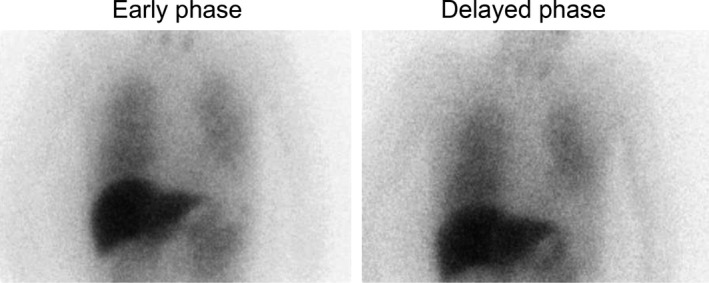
Results of iodine‐123‐metaiodobenzylguanidine scintigraphy on day 64 in a 72‐year‐old man who ingested and survived a lethal dose of diethylene glycol. Cardiac iodine‐123‐metaiodobenzylguanidine uptake was significantly reduced both in early and delayed phases.
